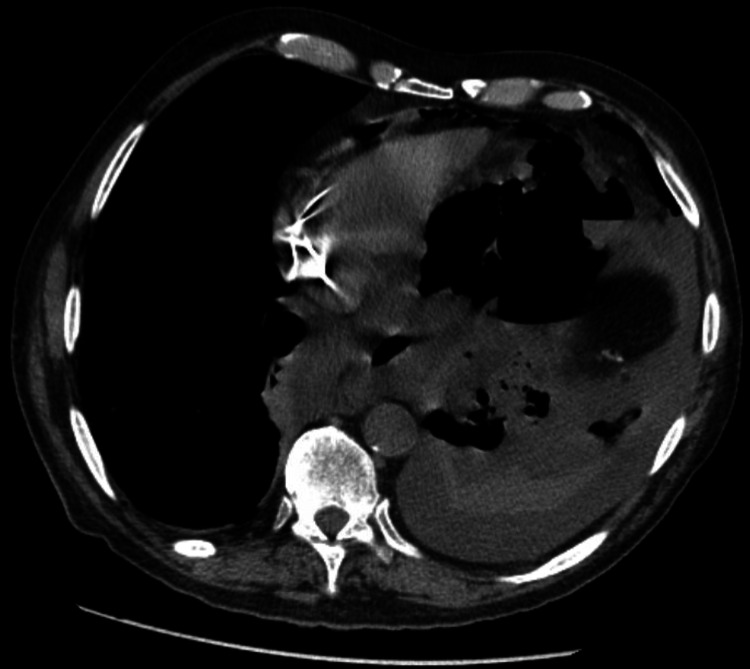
Axial chest CT scan from 2024 CT scan reveals a third gastropleural fistula accompanied by a large fluid collection in the left hemithorax.
